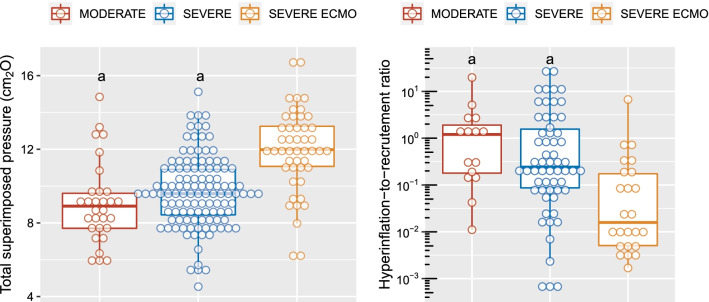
Total superimposed pressure at PEEP 5cmH_2_O and hyperinflation-to-recruitment ratio as a function of ARDS severity. The total superimposed pressure is the hydrostatic pressure superimposed in the most dorsal level of the lungs, computed with CT, assuming that pressure is transmitted through the lung parenchyma as in a fluid. Data points are individual values in each lung for total superimposed pressure and individual values in the whole lung for hyperinflation-to-recruitment ratio. ^a^, *p* < 0.05 vs severe ARDS under ECMO.ARDS, acute respiratory distress syndrome, CT, computed tomography, ECMO, extracorporeal membrane oxygenation, MODERATE, moderate ARDS, PEEP, positive end-expiratory pressure, SEVERE, severe ARDS without ECMO, SEVERE ECMO, severe ARDS under ECMO
